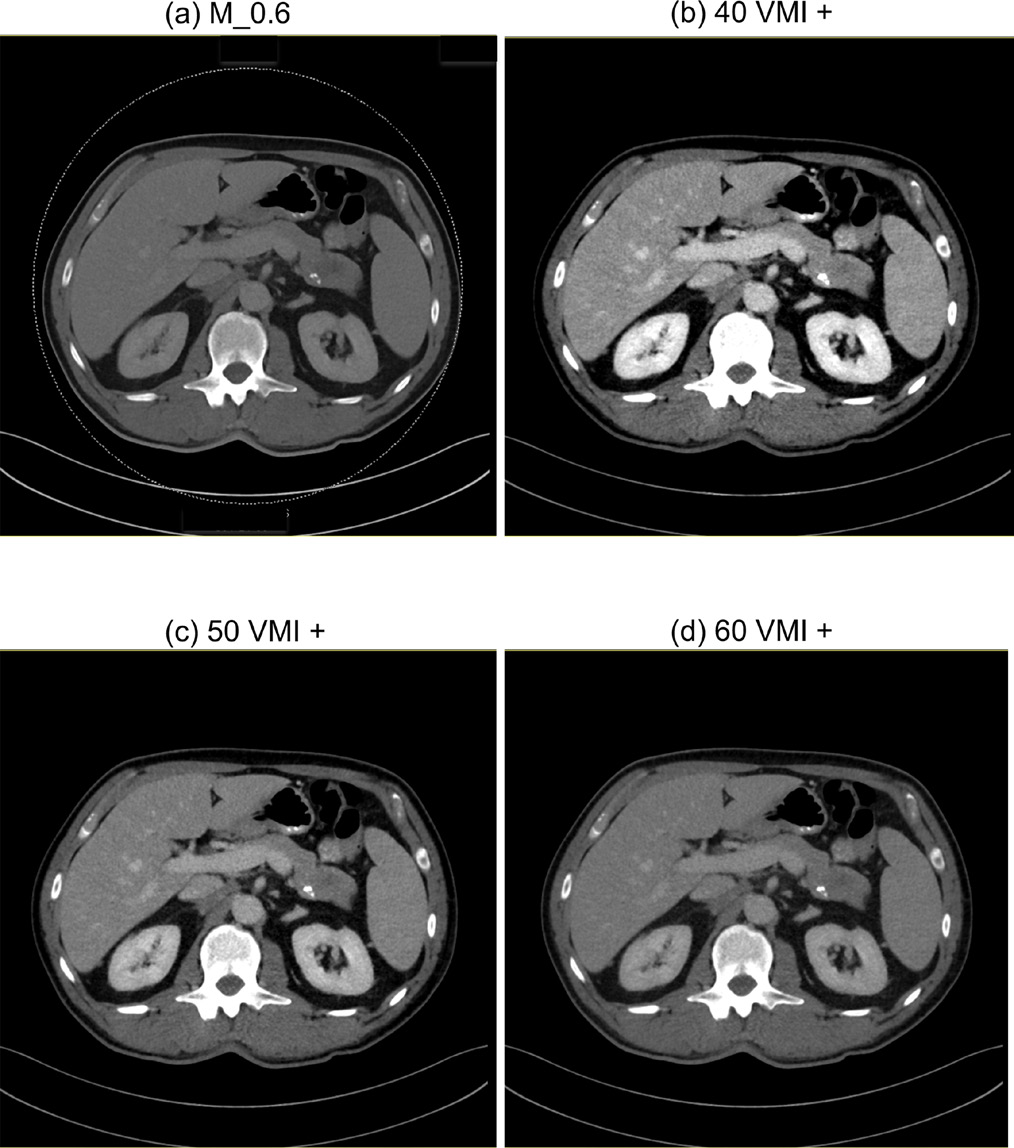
Axial DE-CT images of a 45-year-old male patient. M_0.6 (a), 40 VMI+ (b), 50 VMI+ (c), 60 VMI+ (d). Window settings in all reconstructions: level, 56 HU; width 342 HU.
